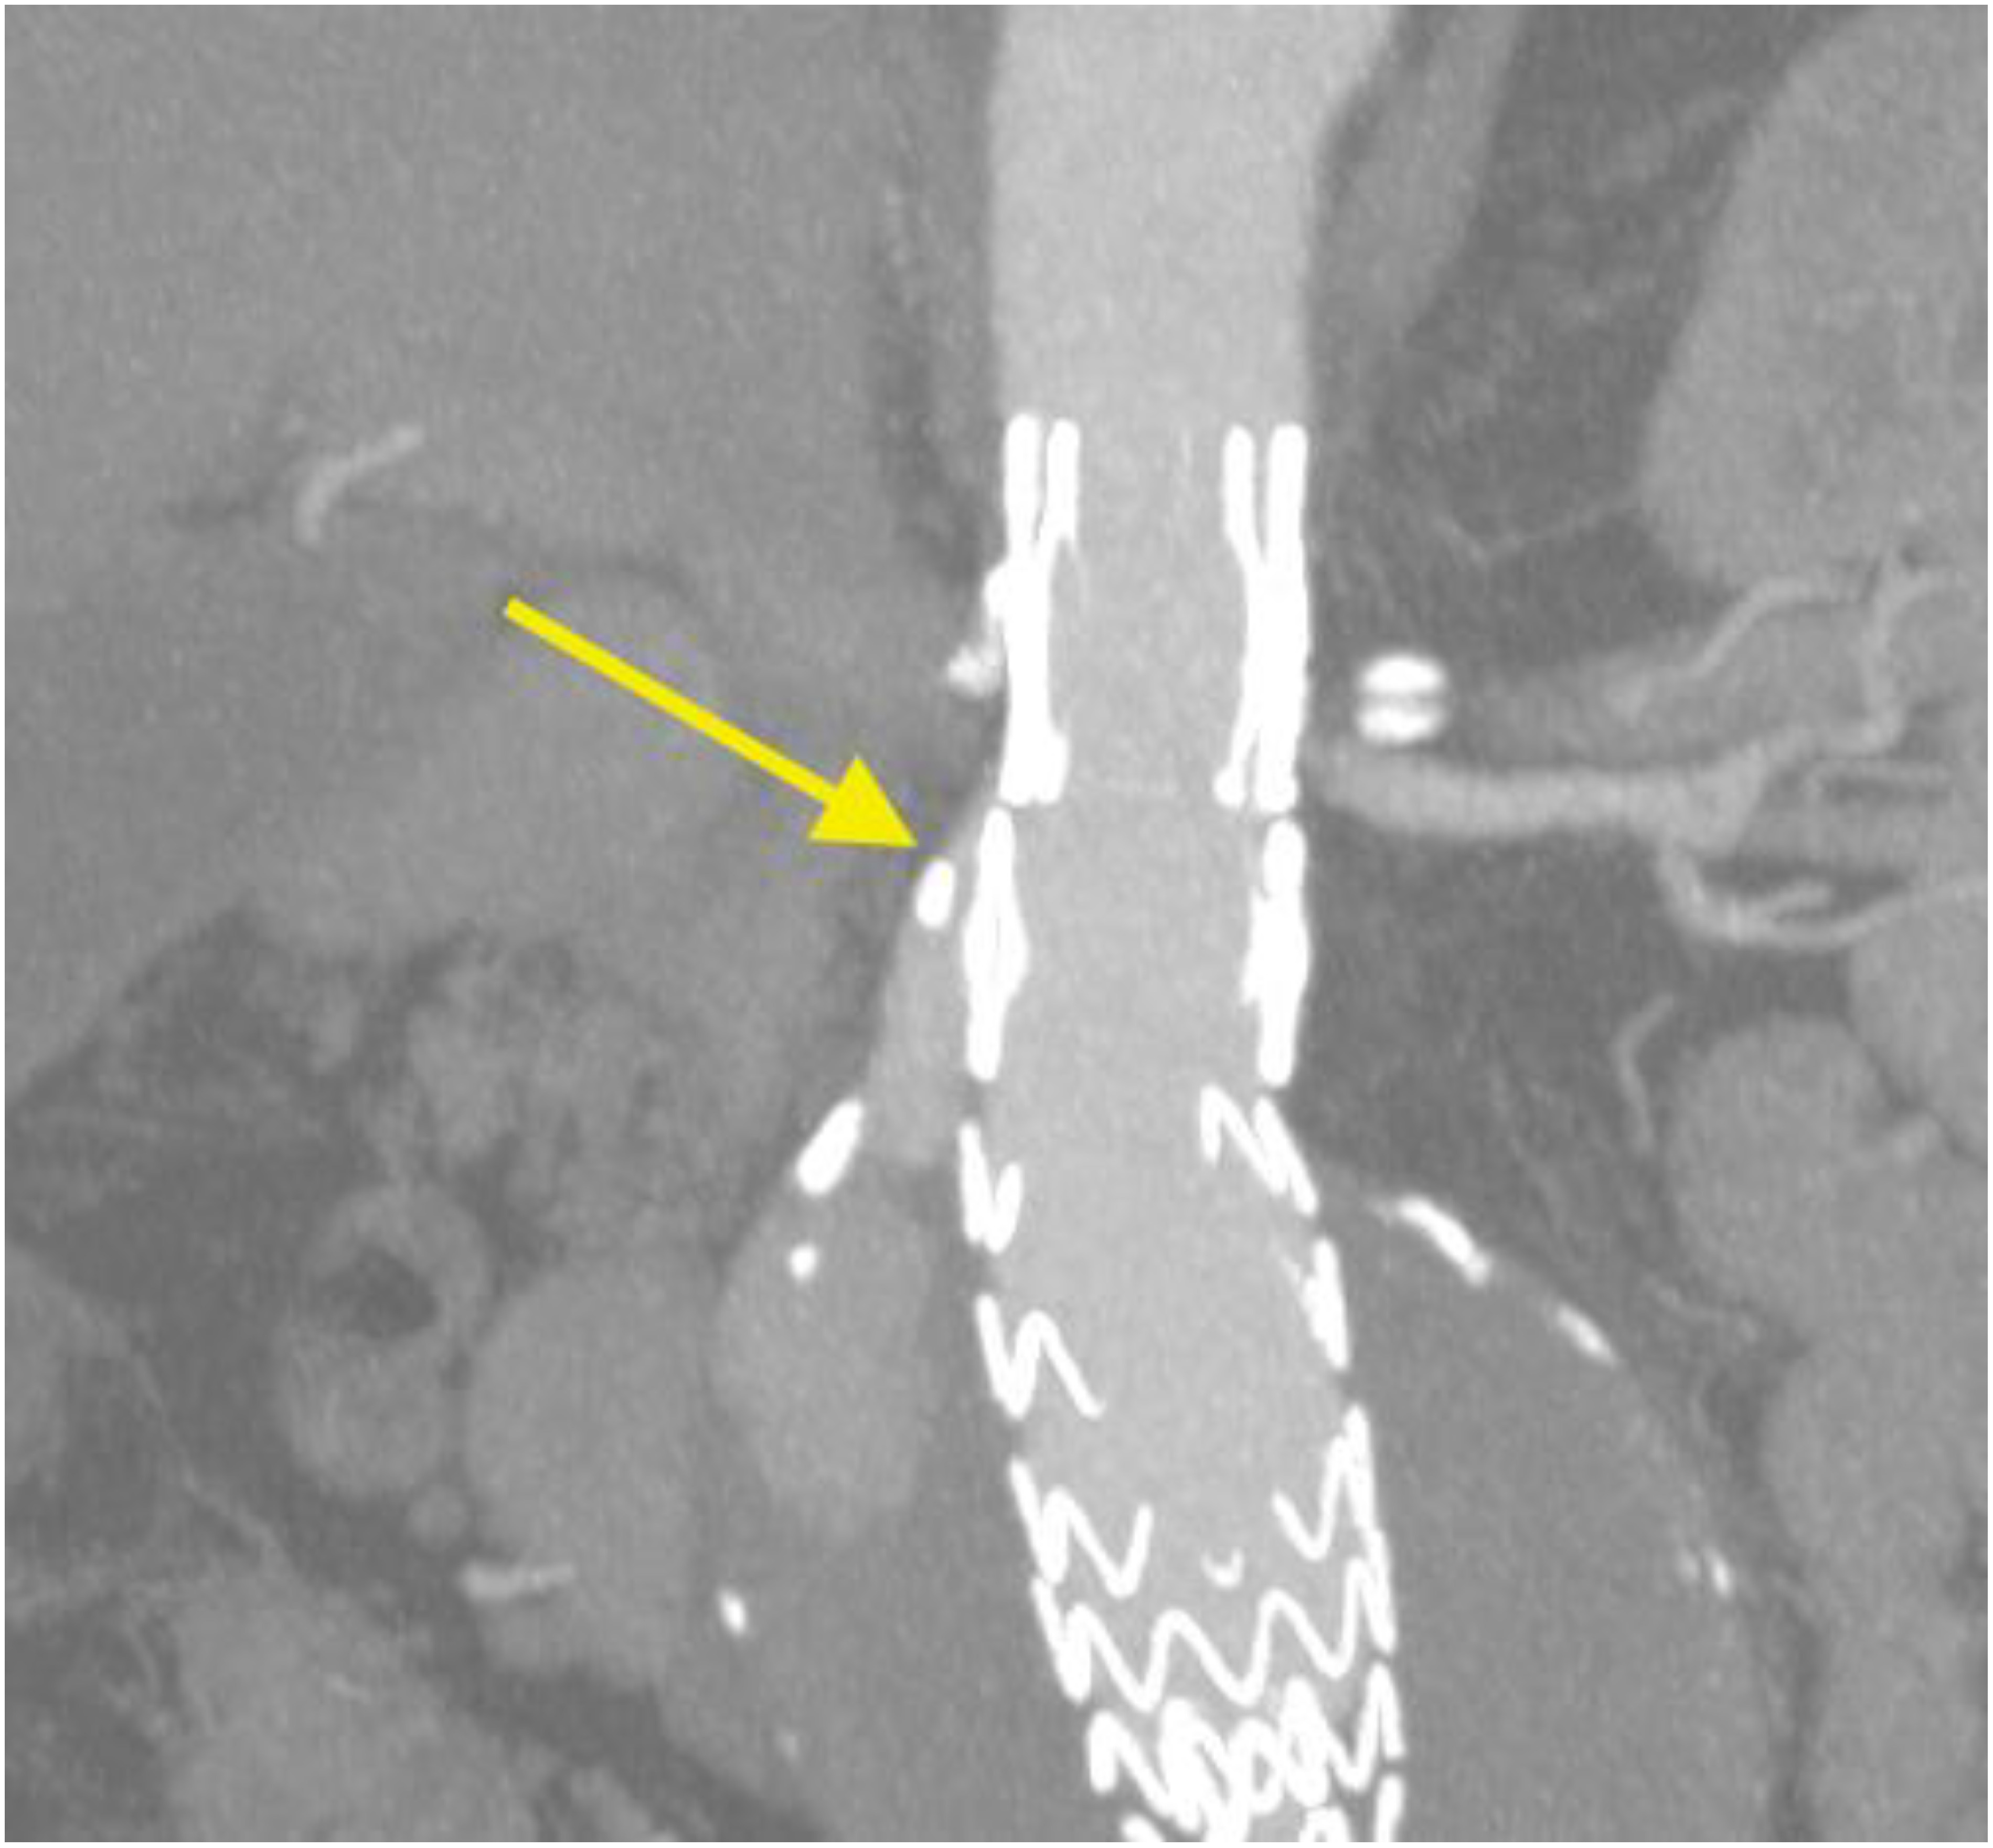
Fig. 2 Preoperative computed tomography angiography of active refractory type 1a endoleak.
